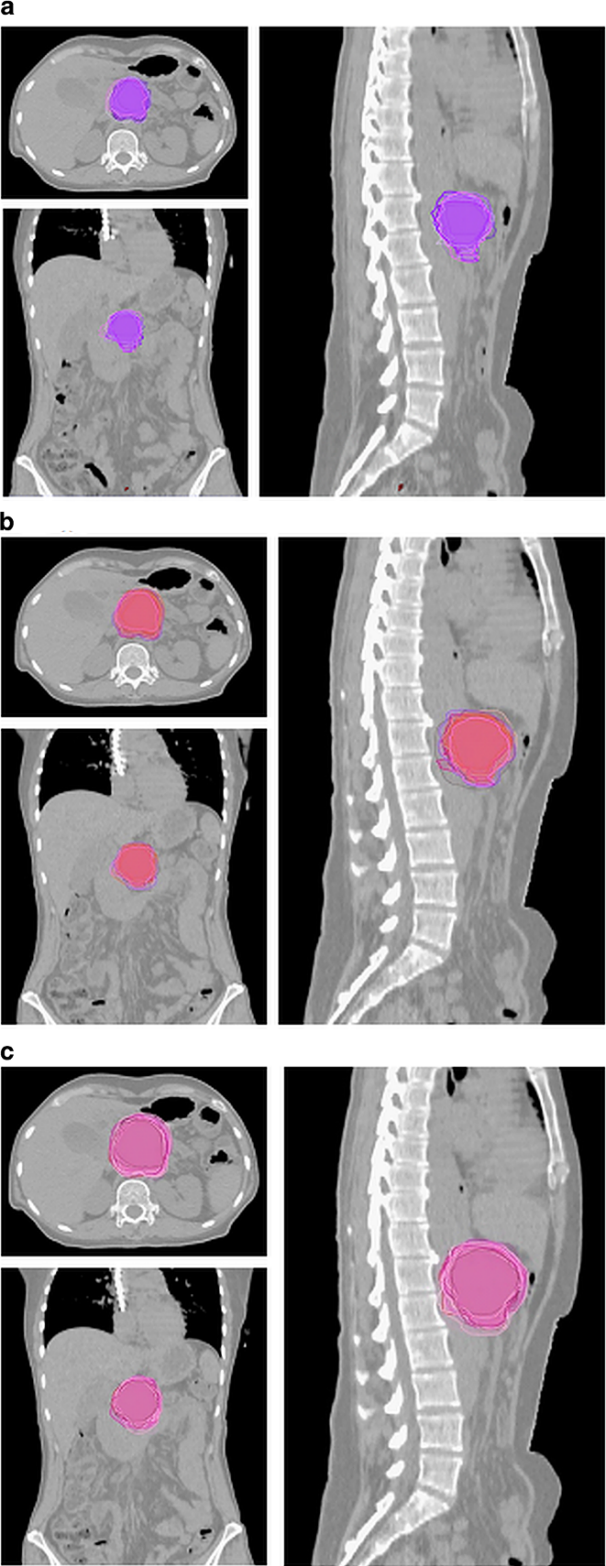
Case 2: patient with progression after initial chemotherapy. Definition of the target volumes based on 4D PET/CT in combination with diagnostic MRI with IV contrast. a GTV, b ITV, c PTV
